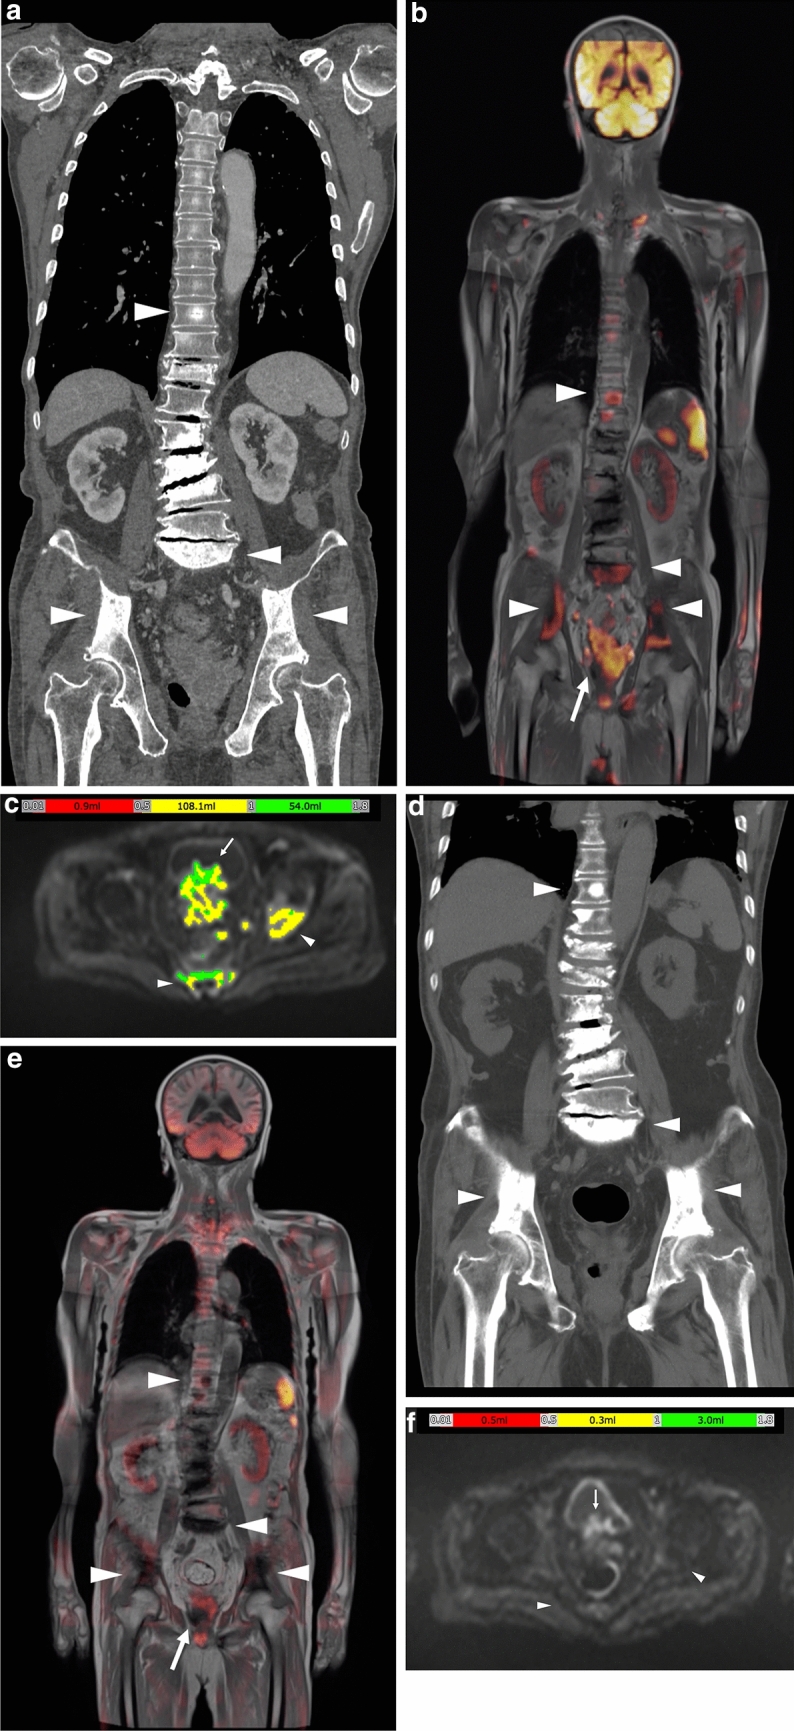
An 85-year-old man who was initially diagnosed with primary unknown multiple bone metastases. a On the coronal reconstructed CT image, sclerotic lesions were observed in the bilateral ilium, sacrum and Th10 (white arrowheads), which were considered osteoblastic metastases. Sclerotic change in the lumbar spine appeared to be degenerative. Following the CT examination, a high serum PSA level (> 5000 ng/mL) was detected. b On the fused coronal DW and in-phase T1W image at a similar level to a, high-intensity areas were shown in the bilateral ilium, sacrum and Th10 (white arrowheads). In addition, the prostate gland was enlarged and appeared as high intensity (white arrow). c BD score composed axial image at the level of pelvis. The total volume of the area with a specific ADC value range (0.01 to 1.8 × 10^–3^ mm^2^/s in the image) is defined as tDV and is subcategorized and denoted by a specific color (0.01–05: red, 0.5–1: yellow, 1–1.8: green). Color-displayed lesions are shown in the left acetabulum and sacrum (white arrowheads). The enlarged prostate gland can also be observed as color-displayed lesions (white arrow). In this case, the tDV was 163.0 mL, and the mean ADC of these lesions was calculated as 0.96 (× 10^–3^ mm^2^/s) by the ADC histogram. d-f. Three months after the image shows in a–c, following combined androgen blockage. PSA decreased to 175.483 ng/mL. d On the coronal reconstructed CT, sclerotic change showed an increase from that in a in the bilateral ilium sacrum and Th10 (white arrowheads). e On the fused image, high-intensity areas in the bilateral ilium, sacrum, and Th10 decreased in intensity (white arrowheads), and the prostate gland decreased in size and intensity (white arrow) from those observed in b. f On the BD score–composed image, the color-displayed area shown in c largely disappeared (white arrowheads and white arrow). tDV markedly decreased to 3.8 mL, and mean ADC value increased to 1.18 (× 10^–3^ mm^2^/s). Osteosclerotic change on d had been considered as the re-ossification after therapy
